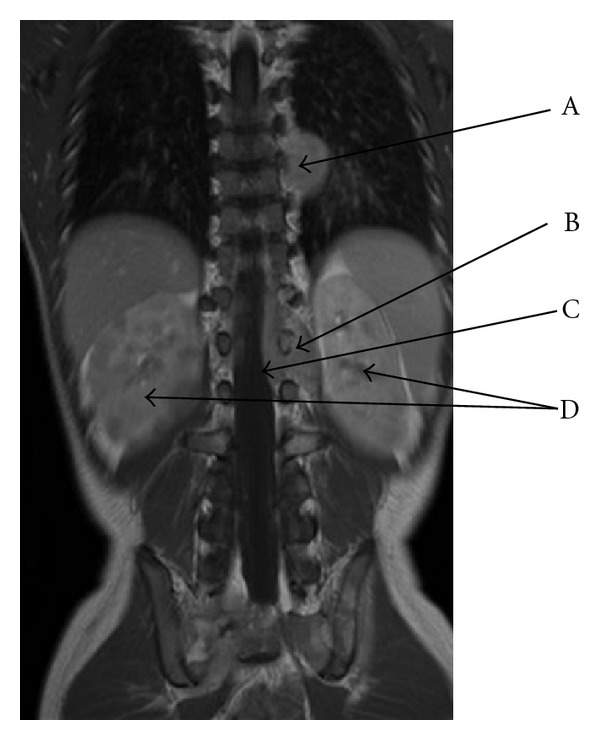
MRI (coronal T1 gadolinium): left dorsal paravertebral tumor (T7–T9) (A) and left dorso-lumbar paravertebral mass (B) with epidural compression (C) through infiltration via intervertebral foraminae (T12–L2). Bilateral nodular nephromegaly (D).
